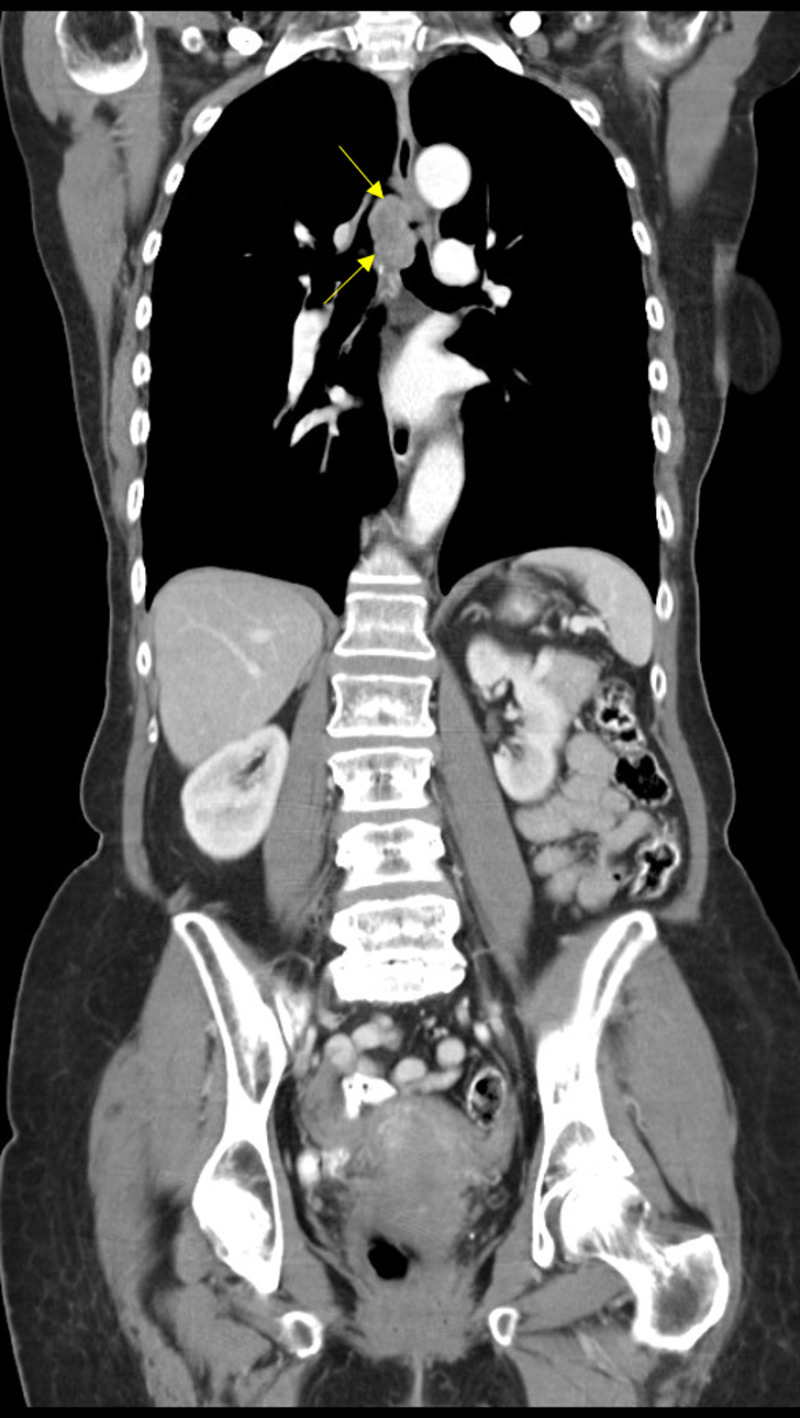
Coronal view of CT Chest showing a 4 cm mass near the trachea.
